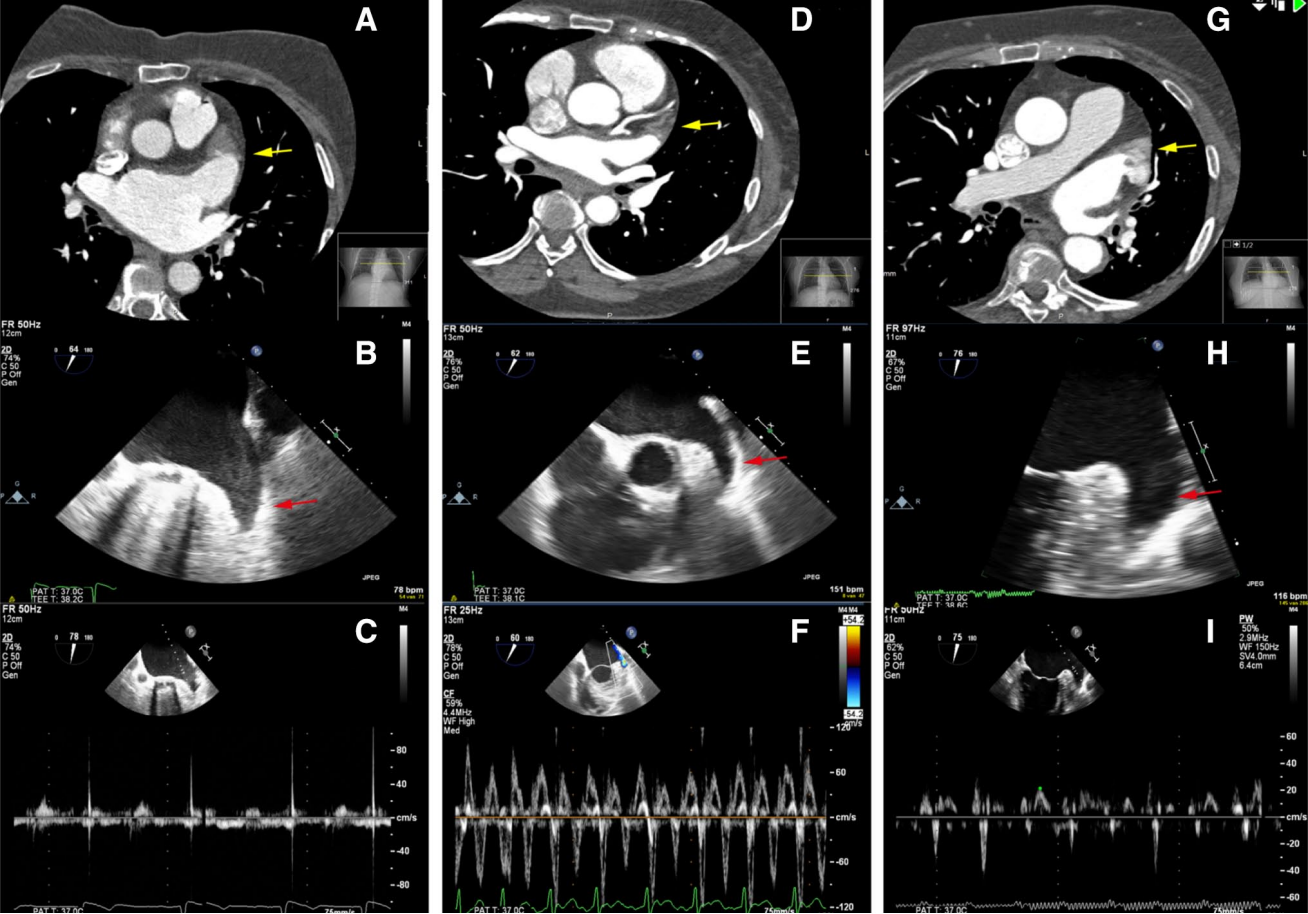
Three patients with false positive CTA scan. LAA filling defects were classified as high (a), intermediate (d) and low (g) risk of thrombus. The patients with a high and low risk filling defect were in AF during performance of CTA, the patient with an intermediate risk filling defect was in sinus rhythm. a CTA in axial view showing LAA filling defect (*yellow arrow*). The area of low attenuation shows a homogeneous aspect, HU 50–70 and a well-defined convex border. b TEE showing severe spontaneous echo contrast (*red arrow*) in the LAA without thrombus. c Low velocity measured by pulse Doppler. d CTA in axial view showing LAA filling defect (*yellow arrow*). The area of low attenuation shows a inhomogeneous aspect, HU 70–110 and a well-defined convex border. e TEE showing no thrombus and no spontaneous echo contrast (*red arrow*). f High velocity measured by pulse Doppler. g CTA in axial view showing LAA filling defect (*yellow arrow*). The area of low attenuation shows a inhomogeneous aspect, HU 150–250 and an ill-defined border. h TEE showing moderate spontaneous echo contrast in the LAA without thrombus (*red arrow*). i Low velocity measured by pulse Doppler
